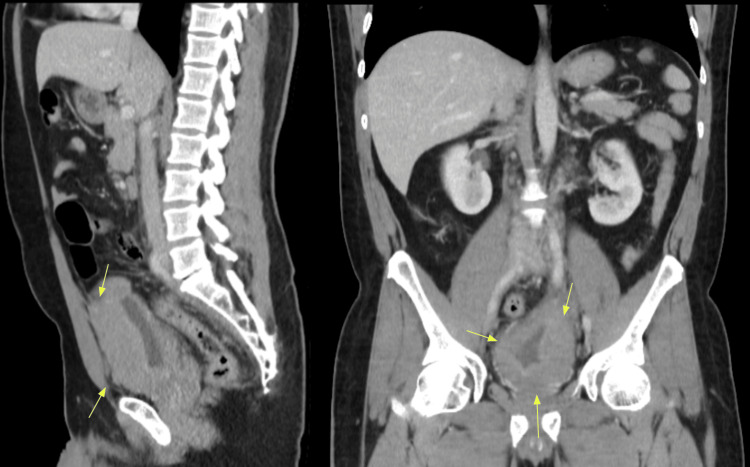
Contrast-enhanced abdominal and pelvic computed tomography. Sagittal (left) and coronal (right) views demonstrating diffuse irregular bladder wall thickening.
